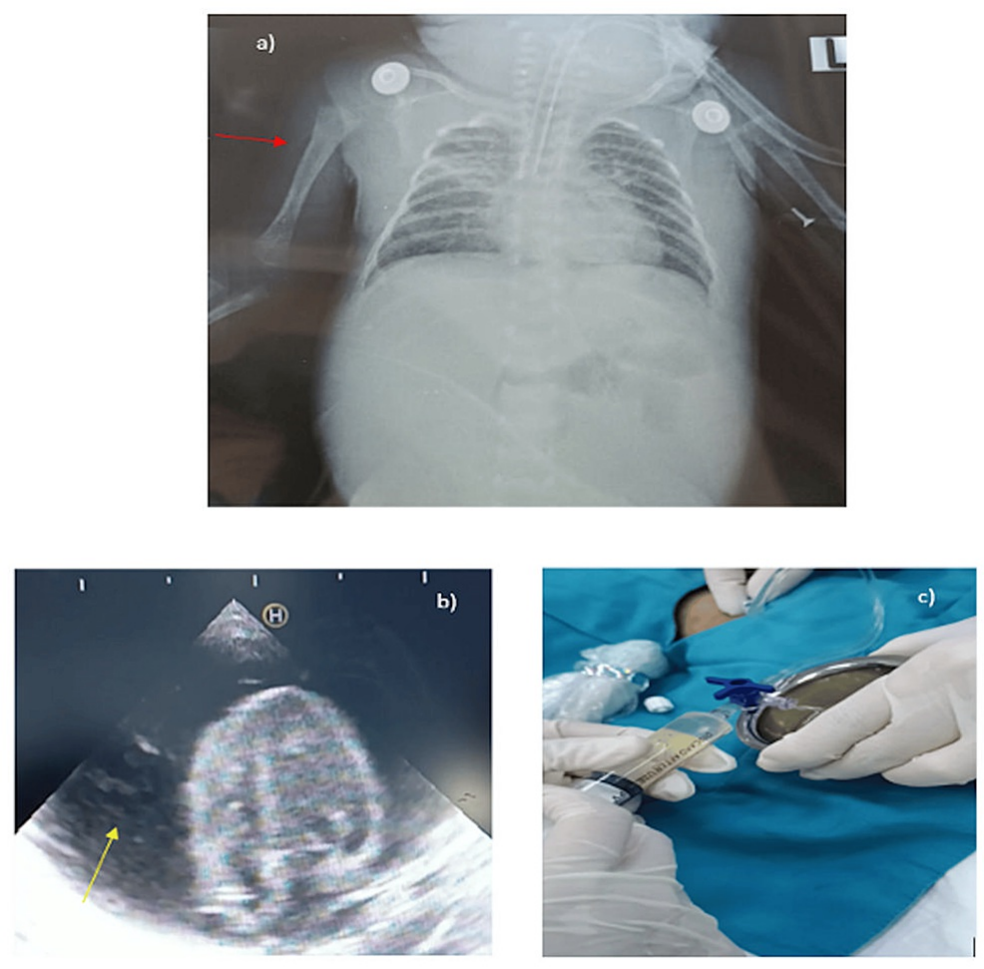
Echocardiogram, chest radiograph, and pericardial fluid of Case 2 a) Chest X-ray performed in a supine position to confirm the PICC position but unable to reveal catheter tip position; b) PCE; c) Pericardiocentesis PICC: Peripherally inserted central catheter; PCE: Pericardial effusion
